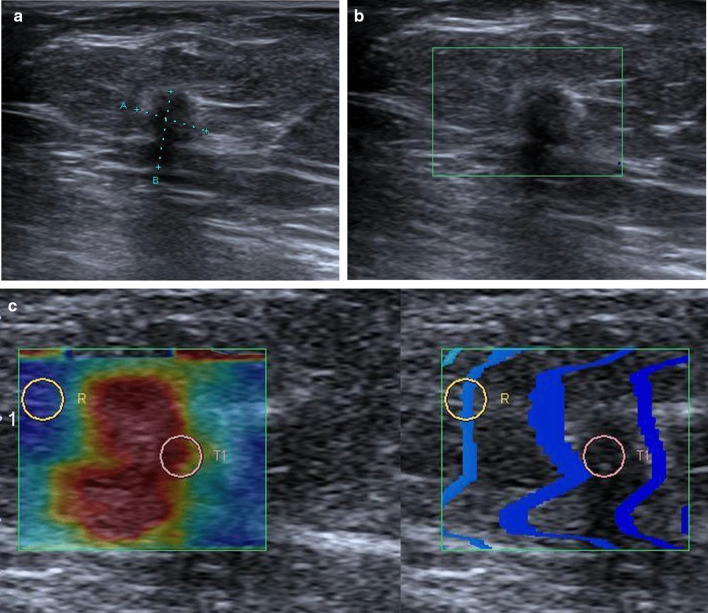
Sonographic findings. a The vertically oriented hypoechoic lesion, irregular in shape with indistinct margins. b No vascular coding in color Doppler image. c High elasticity features with shear wave elastography
